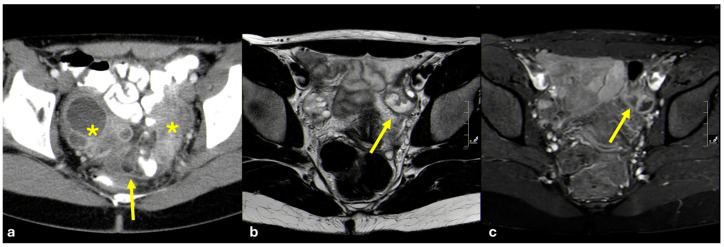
Pelvic inflammatory disease (PID). Two young women with a long history of pelvic pain and purulent vaginal discharge. In the first case, portal venous phase abdominal CT is performed (a) and shows the increased attenuation of the pelvic fat, peritoneal fluid with peritoneal enhancement (arrow), and complex solid-cystic-enhancing masses in both adnexal regions (asterisks); Chlamydia trachomatis is detected as the causal agent. In the second case, pelvic MRI is performed. On T2-weighted axial images (b), left tubal distention with thickened walls (arrow) is appreciable. On contrast-enhanced fat-saturated T1-weighted axial images (c), increased enhancement of the peritoneal spaces as well as of the left salpinx (arrow) is evident. The etiology in this case is polymicrobial.
